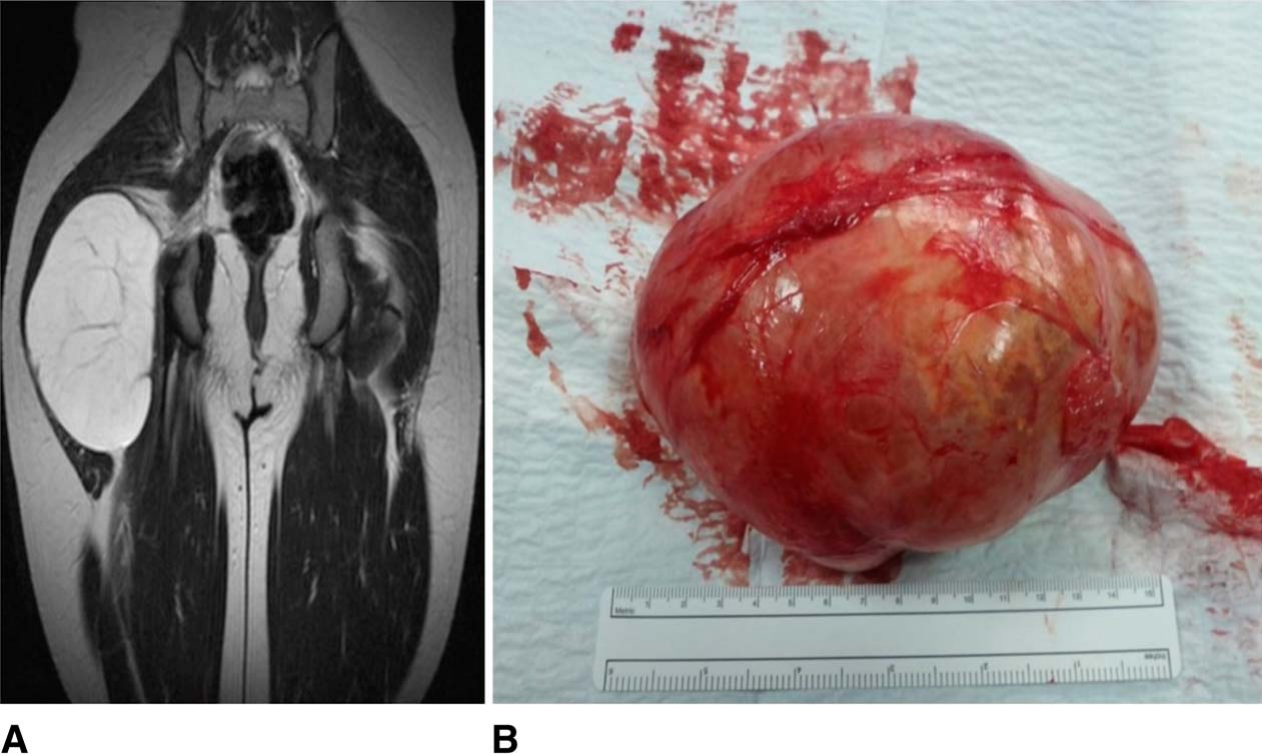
A, Pelvic MRI, T2 sequence, coronal. Close to the right greater trochanter, massive hyperintense lesion localized between the gluteus medius and the gluteus maximus. The lesion compresses the gluteus maximus on the right. B, Intraoperative appearance of the lesion.
